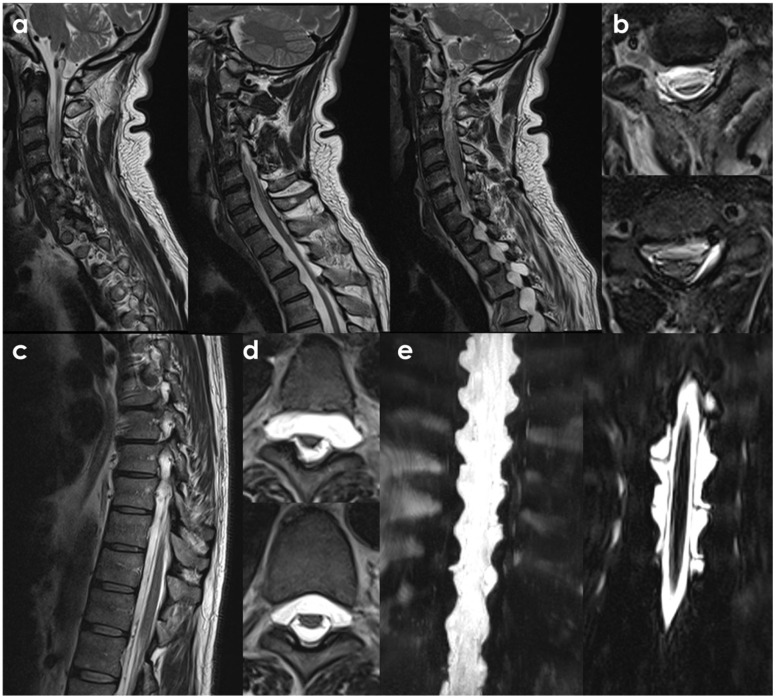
Spine MRI of a patient with brachial amyotrophy with a progressive course within few years after a major trauma with brachial plexus roots avulsion on the right side about 20 years before. Panel (a,b) are sagittal and axial (cervical level) T2W images, showing VLISFC and markedly reduced section of the spinal cord. Panel (c,d) show the corresponding sequences and findings in the dorsal segment. Panel (e) shows MyeloMRI with extensive VLISFC. SIH: spontaneous intracranial hypotension; VLISFC: ventral longitudinal intraspinal fluid collection.
